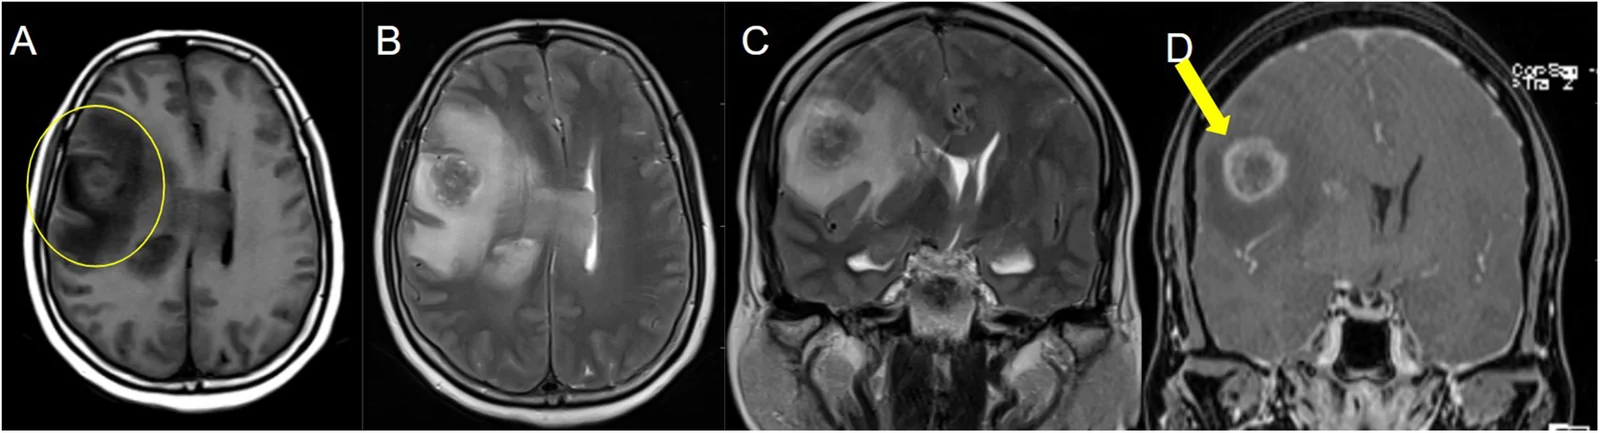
Brain metastases. (A) Axial T1-weighted image shows a necrotic, T1 hypointense lesion in the right frontal lobe with extensive surrounding vasogenic edema (yellow circle). There is significant mass effect on adjacent structures with leftward midline shift. A smaller lesion is noted along the posteromedial aspect of the primary mass. (B) Axial T2-weighted image demonstrates a predominantly T2 hyperintense lesion with extensive surrounding hyperintense vasogenic edema. (C) Coronal T2-weighted image better delineates the degree of mass effect and leftward midline shift. (D) Coronal T1-weighted post-contrast image demonstrates irregular thick peripheral ring enhancement of the dominant lesion (yellow arrow), with a smaller enhancing lesion medially suggestive of metastatic disease.
